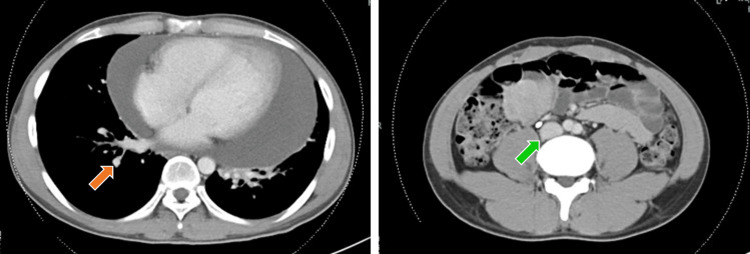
Contrast-enhanced CT of the chest Chest CT showing that the pulmonary emboli (orange arrow) and DVT (green arrow) disappeared on day 84 after the start of entrectinib. CT: computed tomography
